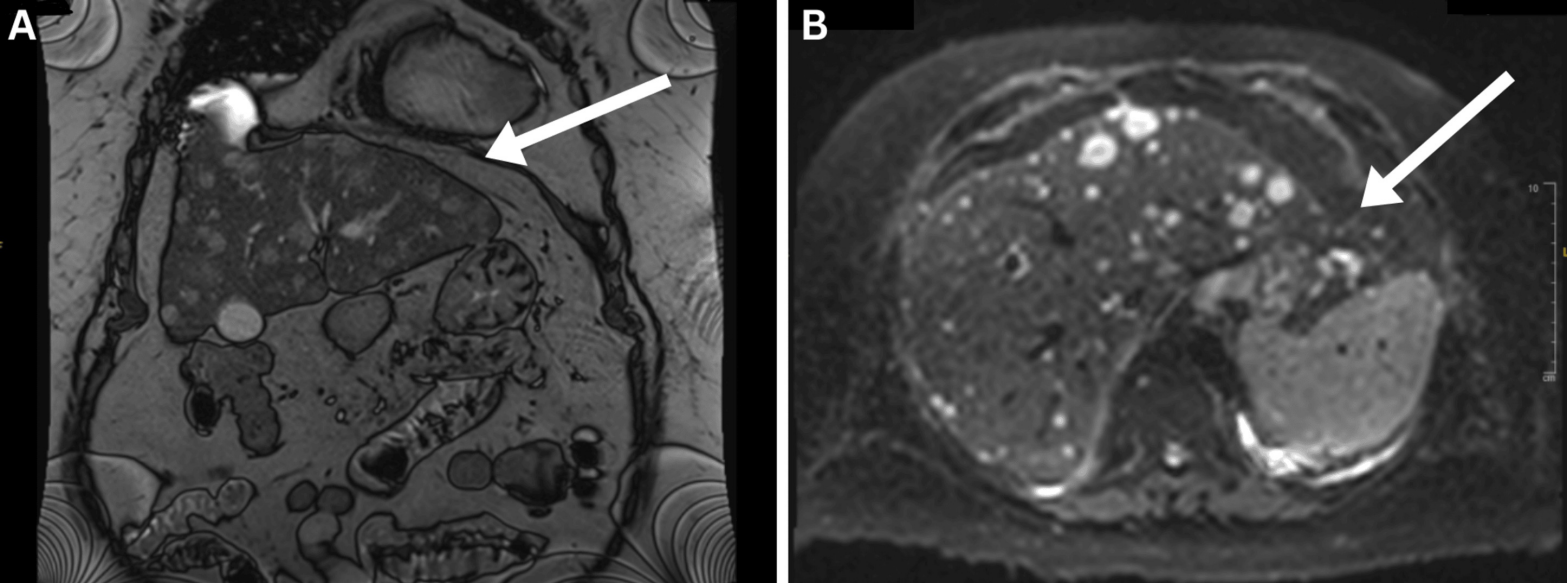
MRI of the liver and pancreas Multiple, innumerable, bilobar hepatic hypovascular metastases seen, with the index lesion in segment 8/5 measuring up to 25 x 21 mm (Image A). Ill-defined pancreatic distal body/tail intermediate T2 signal lesion measuring 5 x 6 cm seen replacing the fatty interdigitation close to the splenic hilum (Image B).
